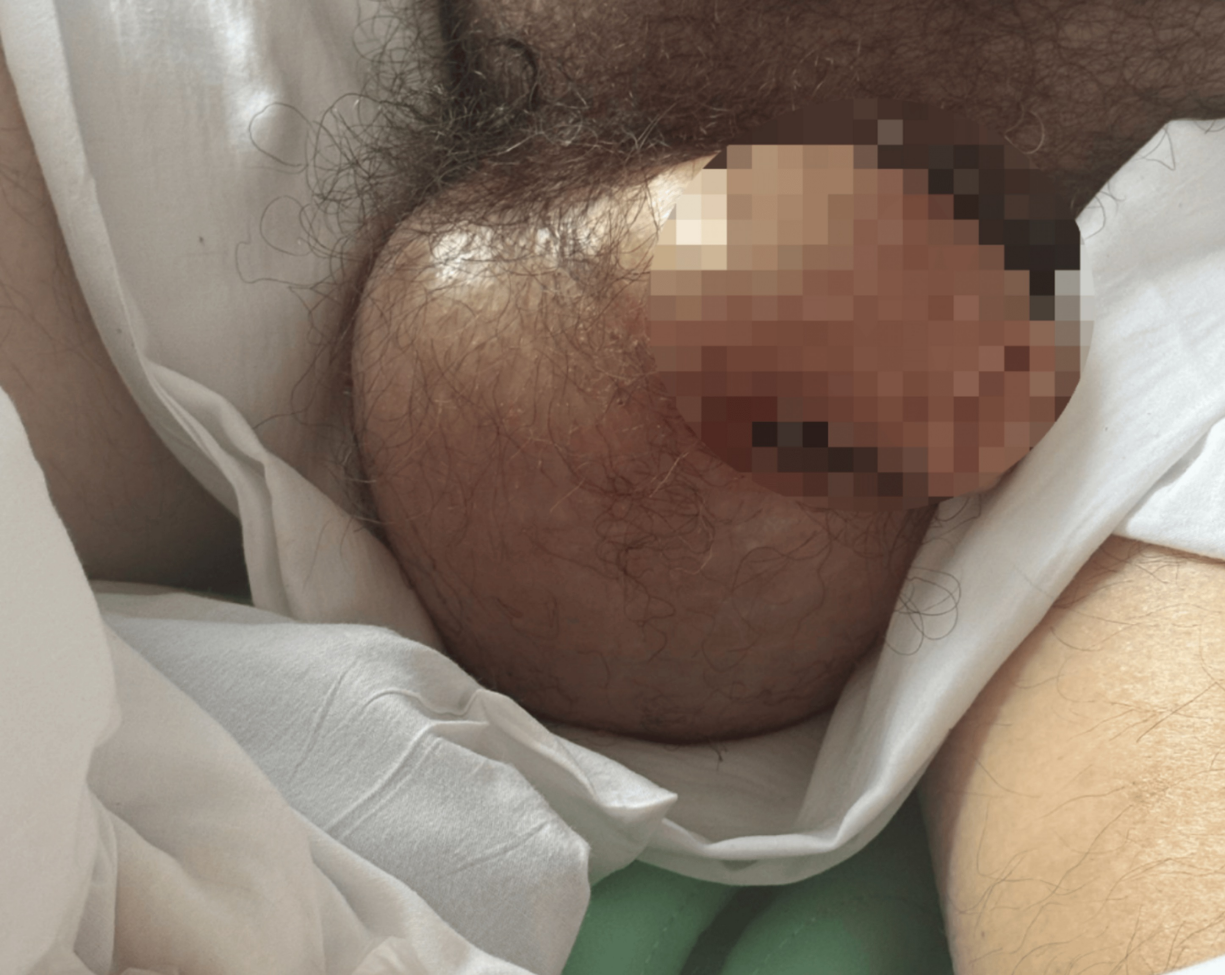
Scrotal edema that developed following diabetic ketoacidosis. The scrotum measured approximately 10 cm in diameter. Ultrasound revealed only edema without acute pathology. Informed consent was obtained from the patient for the publication of the image.
